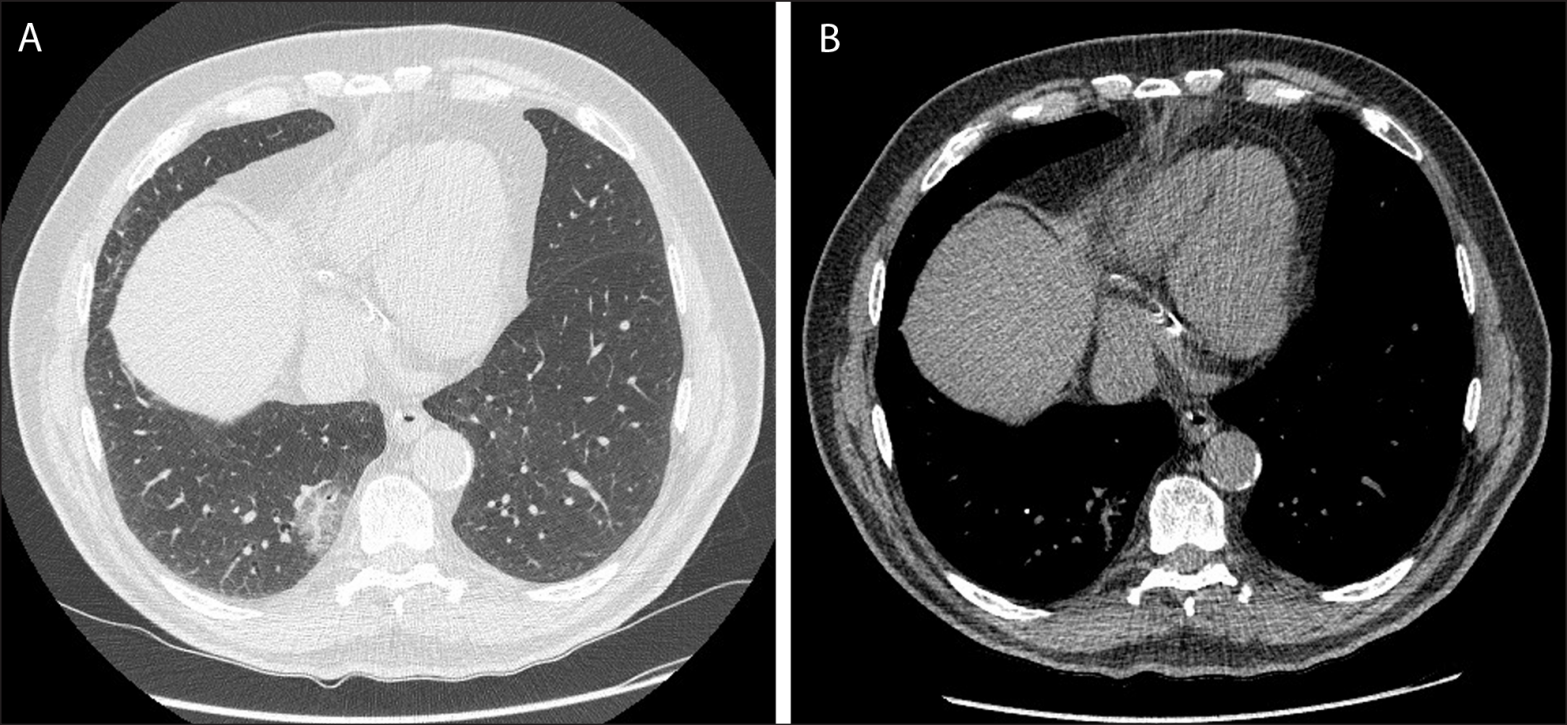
Non-contrast-enhanced CT image of the chest. Axial image in lung window setting (A) shows a well-delineated subsolid nodule in the paravertebral region of the right lower lobe. The lesion has a predominant ground glass appearance. A more prominent vessel is running through the lesion, but in mediastinal setting (B) no clear solid components can be delineated.
